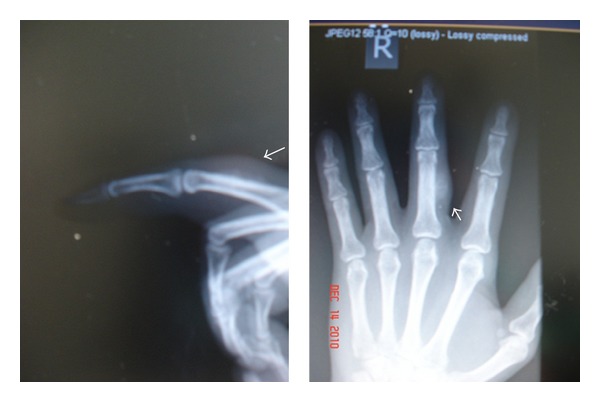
Anteroposterior and lateral views of plain radiographs taken eight weeks after injury. There is a calcified bony tissue.
